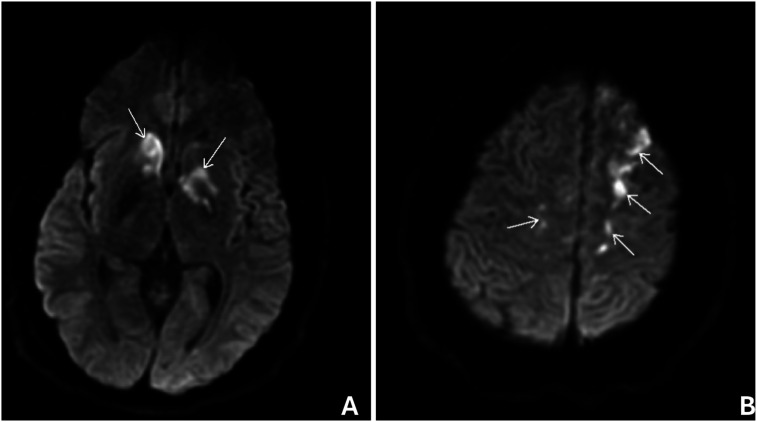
Axial diffusion-weighted magnetic resonance imaging of the brain. The arrows indicate areas of hyperintensity, representing restricted diffusion. These findings are consistent with acute to subacute ischemic infarcts involving the bilateral basal ganglia (A) and frontal lobes, with greater involvement of the left hemisphere (B)
